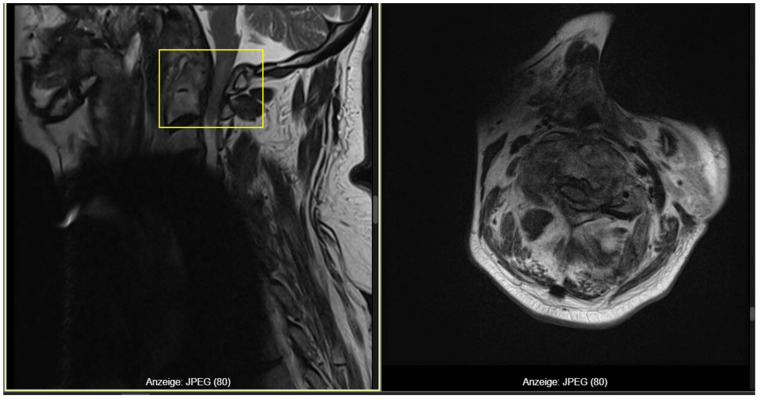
Follow-up MRI-T2 revealing C1–C2 stenosis with myelopathy (frame).
